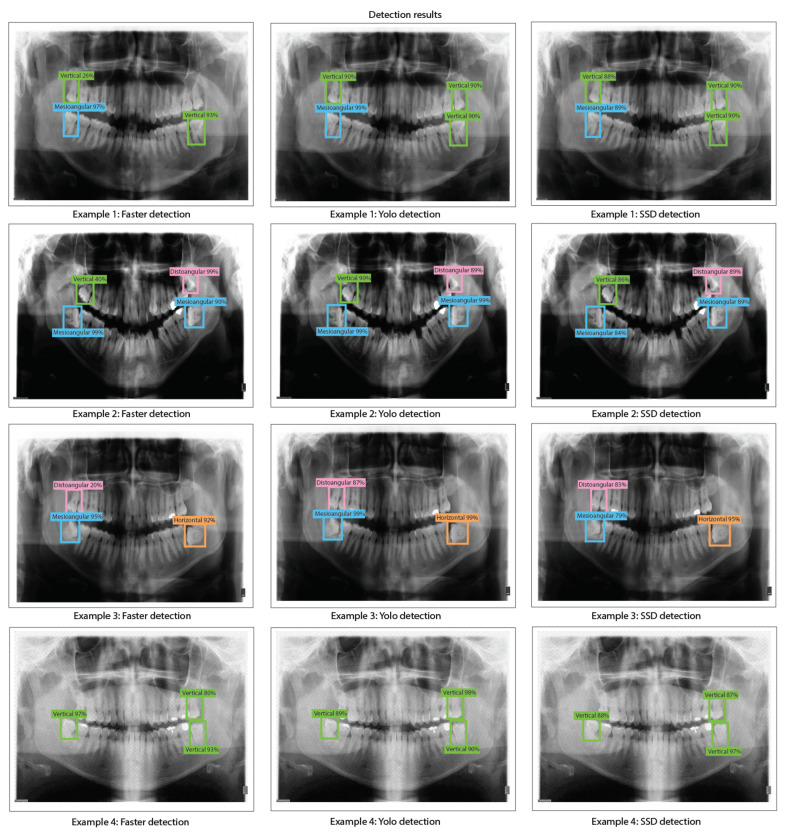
Third molar angle detection in dental panoramic X-rays with ResNet-50 as feature extractor for Faster R-CNN, YOLO V2, and SSD.
